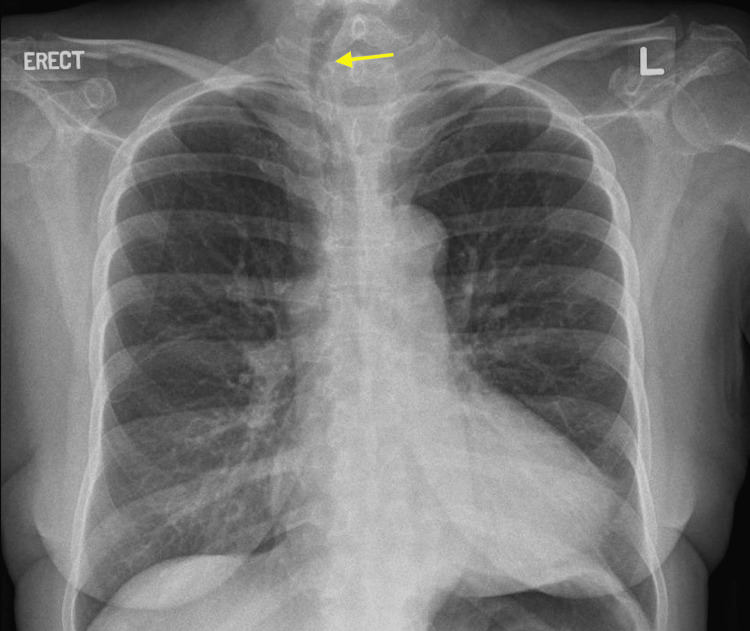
Chest radiograph image showing tracheal deviation toward the right on admission suggestive of an underlying left thyroid goiter.
